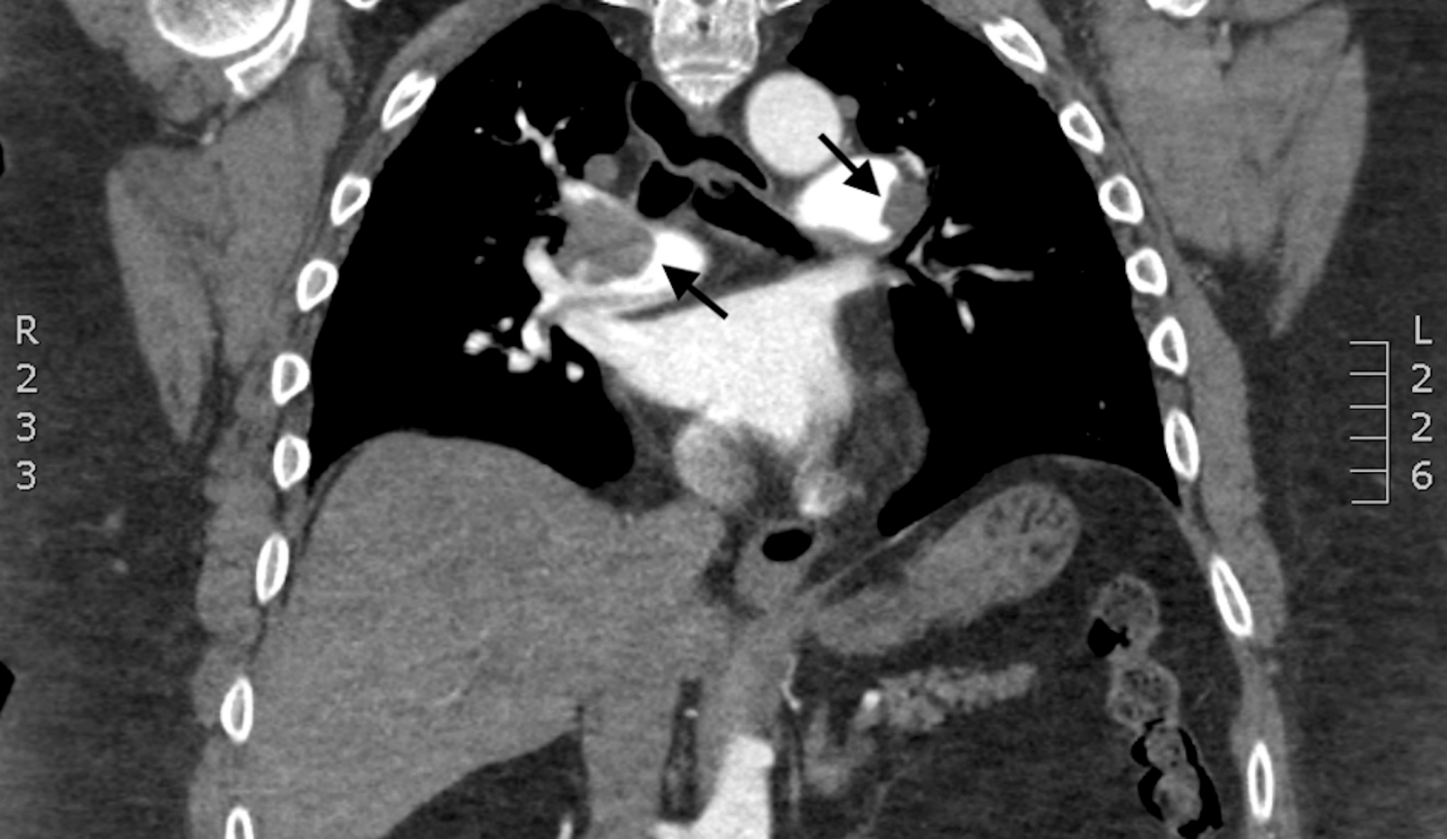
CTA coronal view of chest demonstrating saddle pulmonary embolisms with a large clot burden Black arrows point to the thromboembolisms in the pulmonary artery. CTA, computed tomography angiography.
